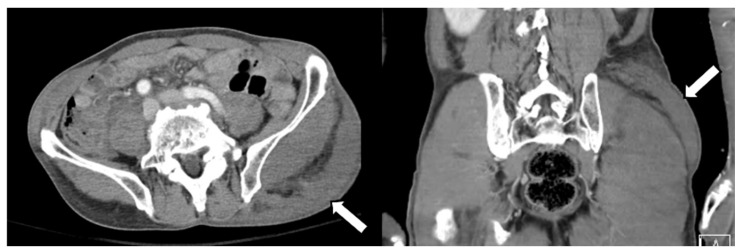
CT scans of the abdomen and pelvis showed an abnormal soft tissue lesion in the left buttock.
